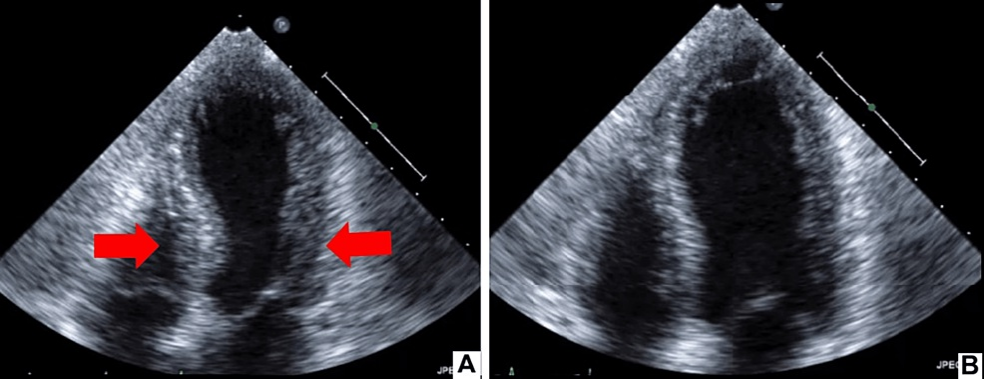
Initial echocardiography Echocardiography of the patient at systole (A) and diastole (B) shows the same motion as the left ventriculography and slightly reduced systolic function (ejection fraction 47%).
